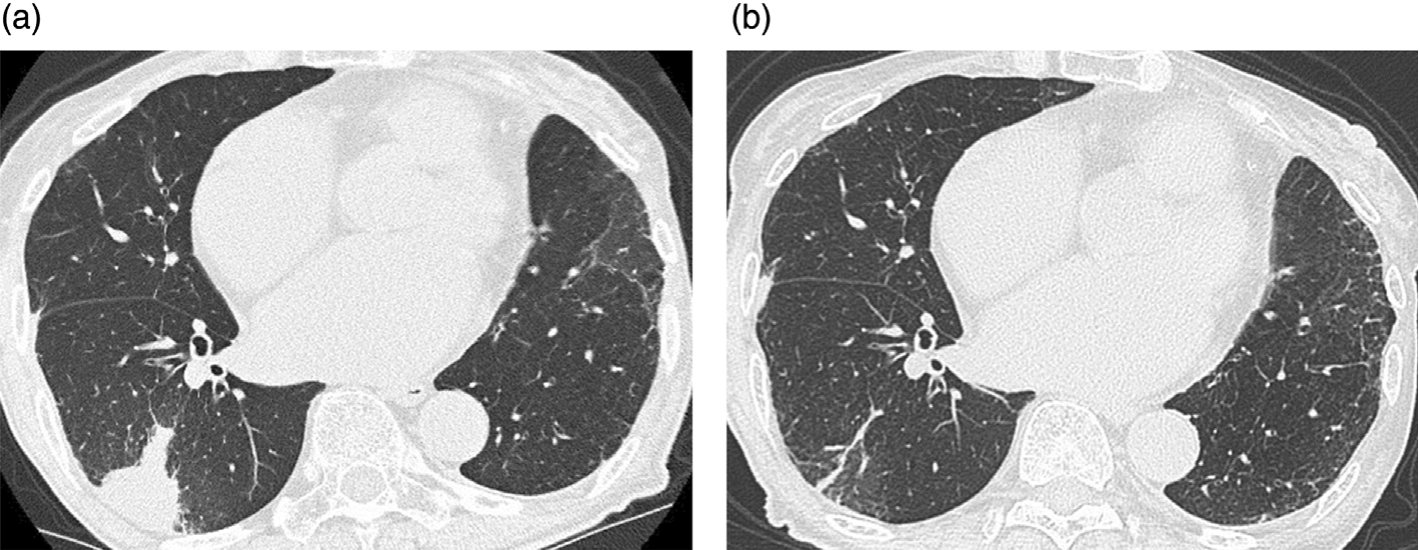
(a) Chest computed tomography (CT) image at the onset of organizing pneumonia. (b) Chest CT image of organizing pneumonia 2 months after the initiation of prednisone.
